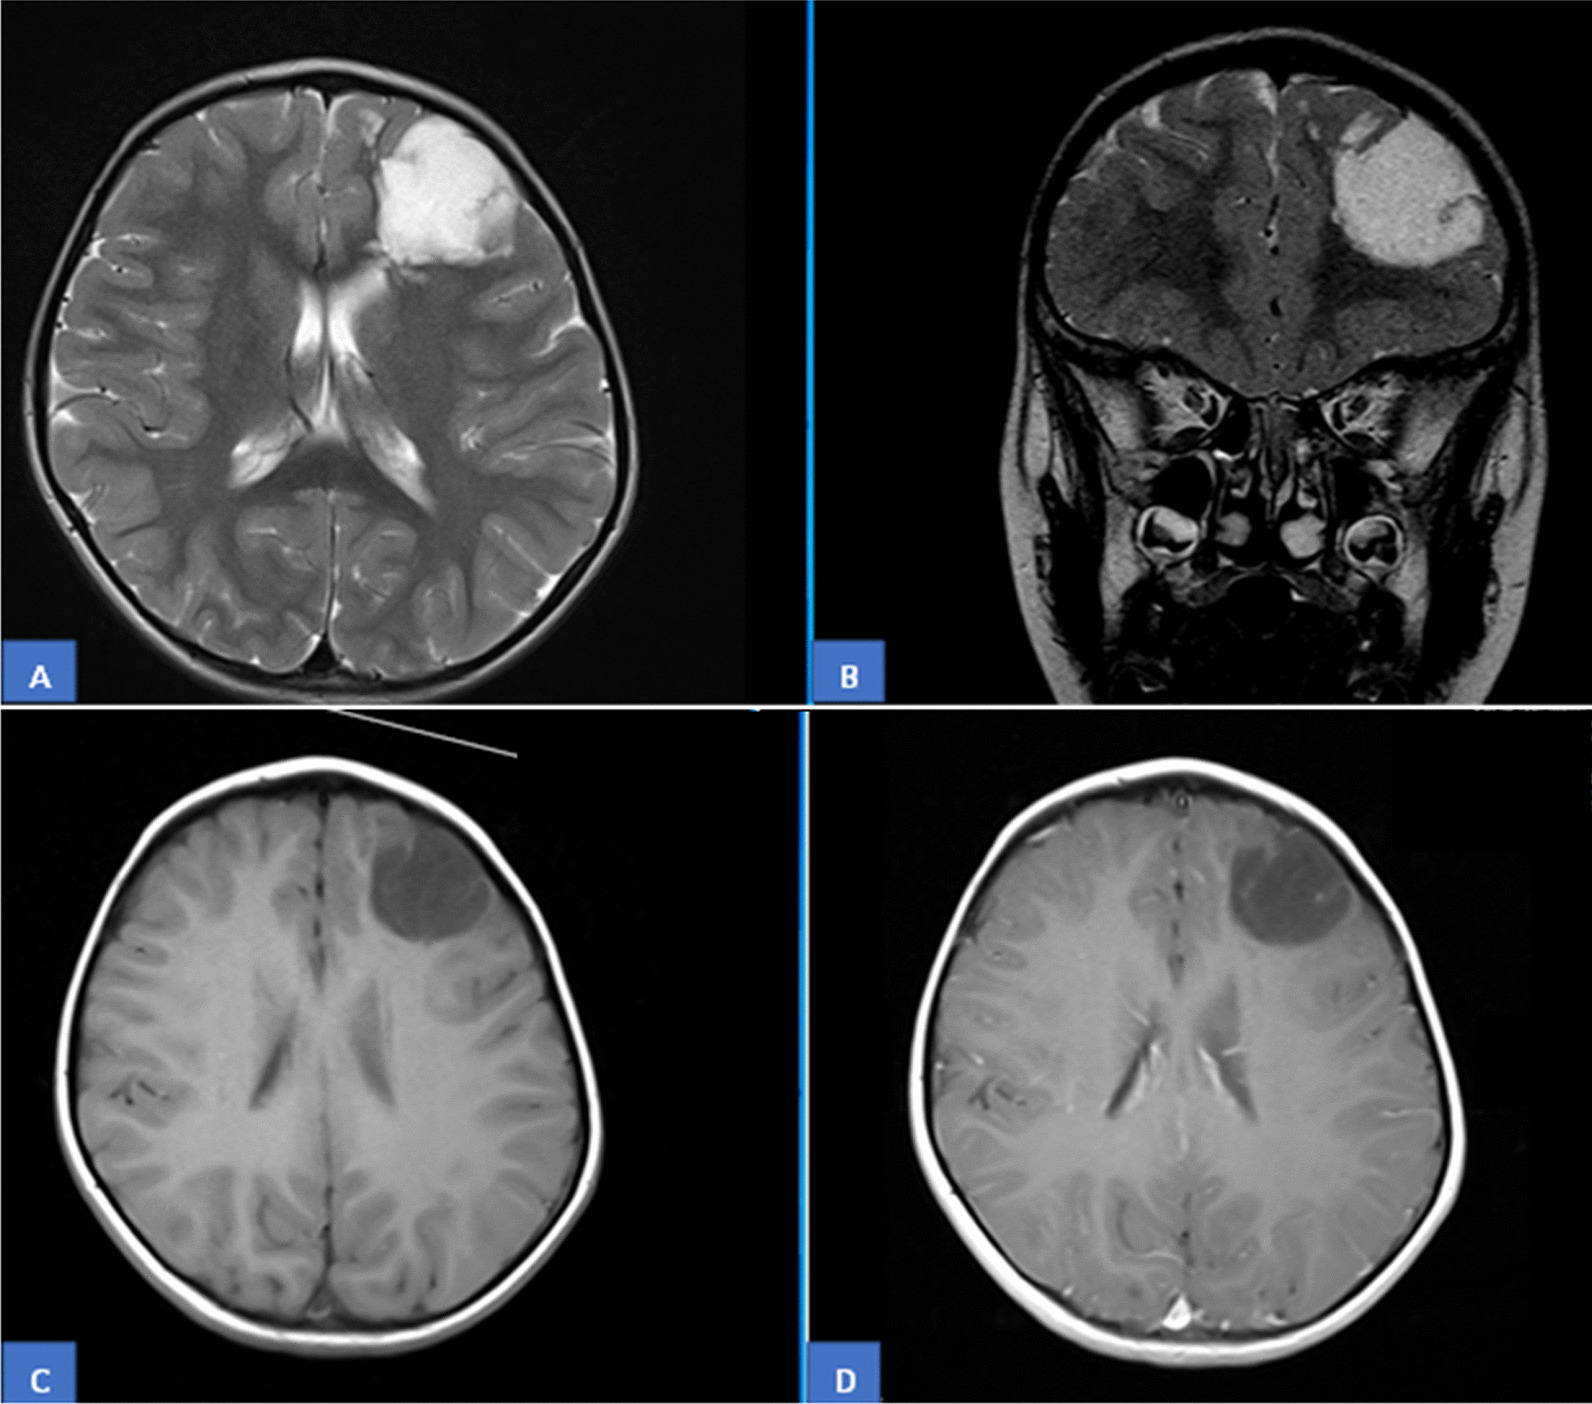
MRI images: A, B A well-defined lobulated T2W cystic cortical and subcortical left frontal lobe lesion is identified. No enhancement is seen on post contrast axial and sagittal images. C, D Peripheral rim sign on FLAIR images. No signal dropout seen on SW1 sequence. Displacement of white matter tracts seen, suggestive of low-grade less aggressive lesion
